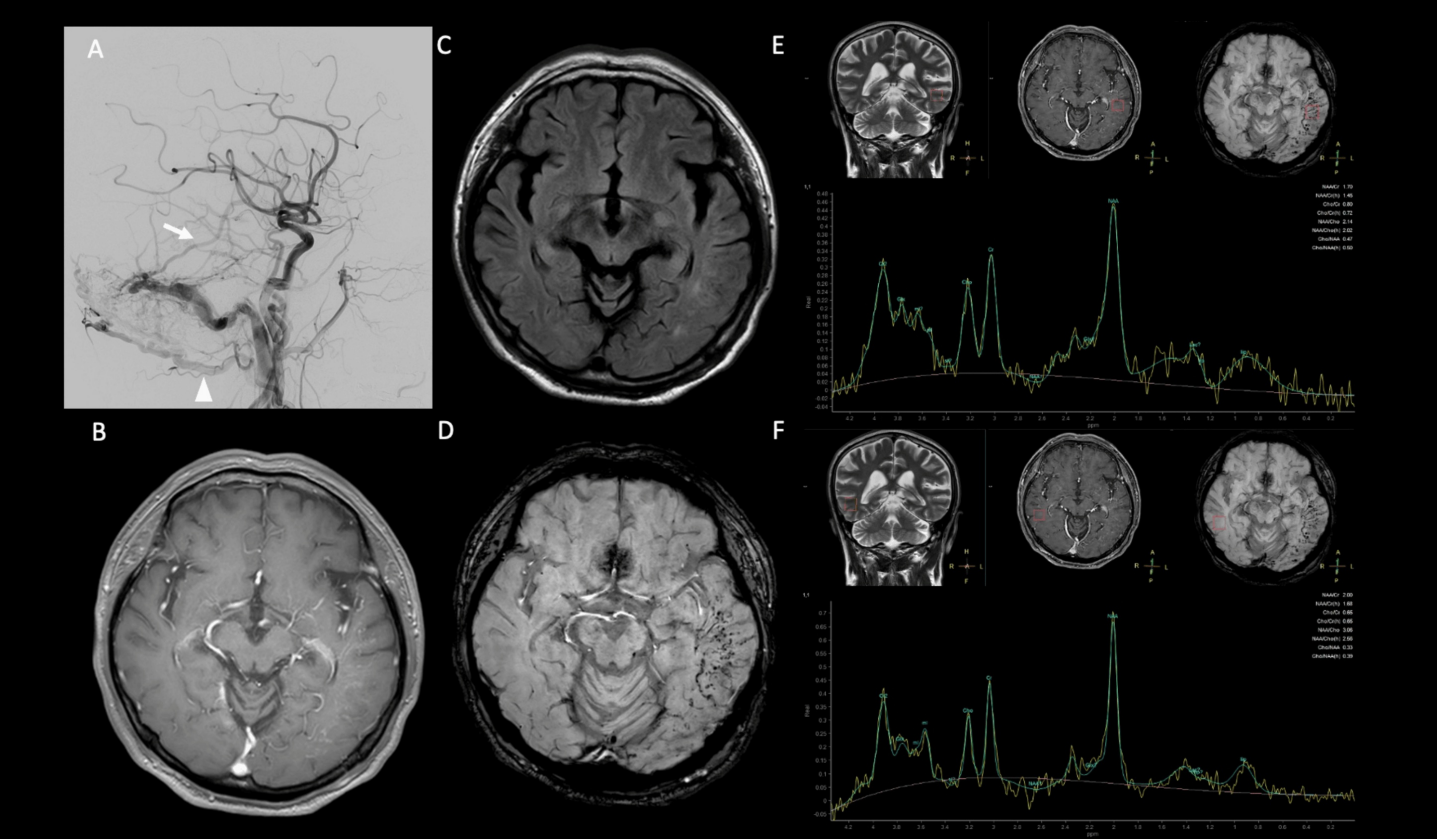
One year after observation. Cerebral angiography shows reflux into the vein of Labbe (white arrow) and the left occipital lobe cortical vein, classified as Cognard IIb (A: lateral view). Slight enhancement is observed in the left temporal lobe (B). FLAIR imaging shows a hyperintense signal in the left temporal lobe (C). SWI reveals venous dilation (D). MRS shows normal levels of both NAA and Lac (E, F) (occipital artery: white arrowhead). FLAIR: fluid-attenuated inversion recovery; SWI: susceptibility-weighted imaging; NAA: N-acetylaspartate; Lac: lactate
